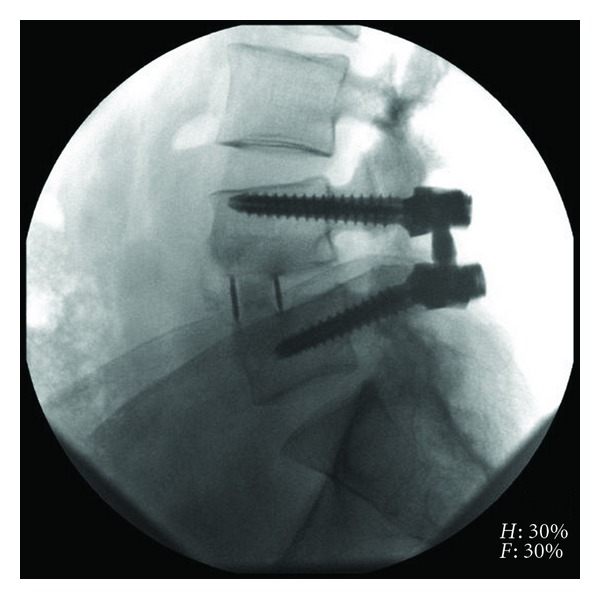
Lateral radiograph of L4-5 extreme lateral interbody fusion with Osteocel Plus at 12-month postoperative.
